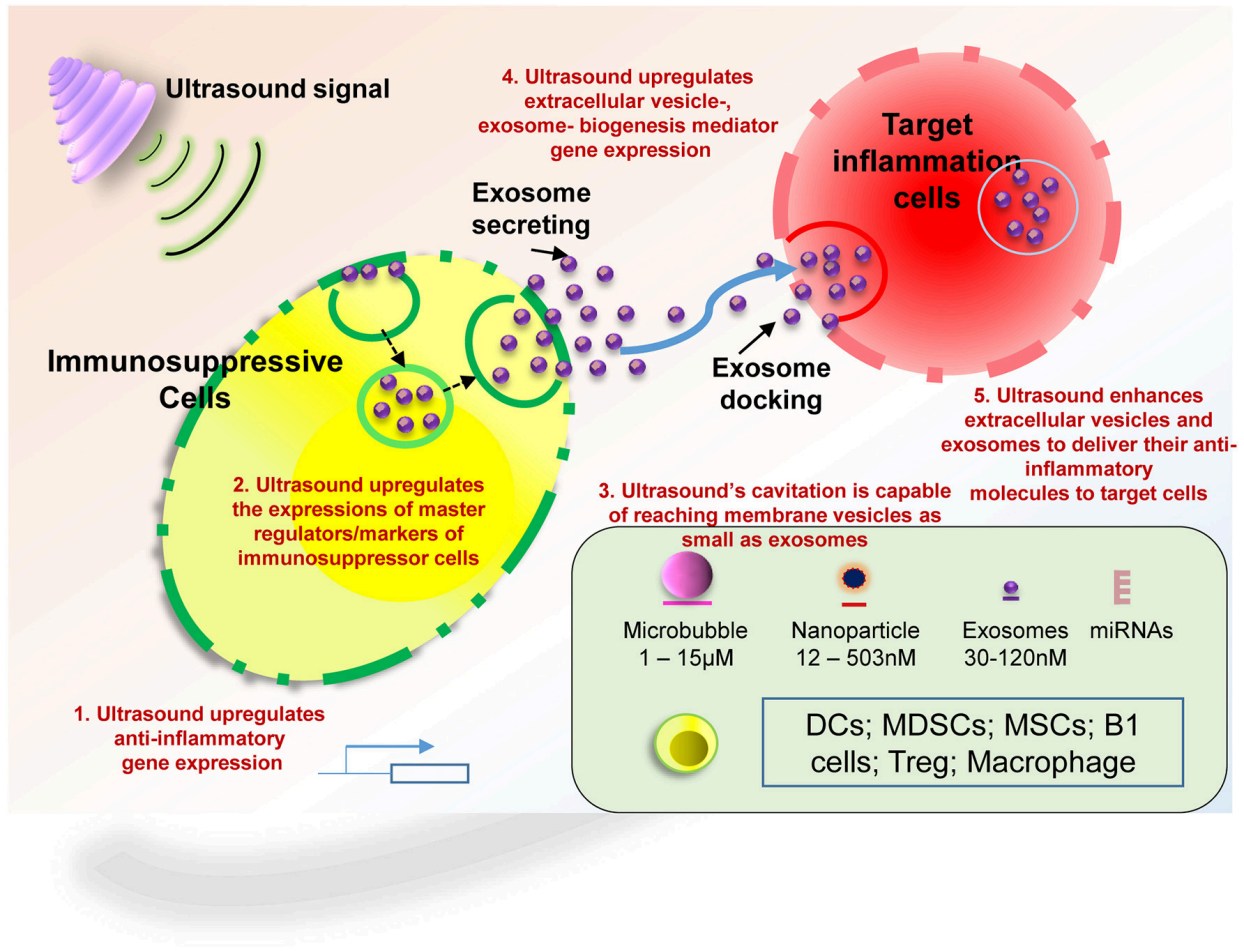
Our novel working model which proposes the potential anti-inflammatory mechanisms utilized by LIUS to exert its beneficial effects. These potential mechanisms are as follows; (1) LIUS upregulates the expression of anti-inflammatory/immunosuppressive genes in the ultrasound-treated cells; (2) LIUS enhances the expression of the master regulators (markers) of immunosuppressor cells; (3) LIUS mediated cavitation is capable of reaching the membrane vesicles as small the expression of extracellular vesicles; and (4) LIUS increases exosome biogenesis and docking mediators, and (5) Ultrasound enhances extracellular vesicles and exosomes to deliver their anti-inflammatory molecules to target cells. Our new findings suggest that LIUS inhibits inflammation via above discussed novel mechanisms to make the target inflammatory cells become less inflammatory. DCs, dendritic cells; MDSCs, myeloid-derived suppressor cells; MSCs, mesenchymal stem cells; B1 cells, CD5+ B1 B cells; Treg, CD4+Foxp3+ regulatory T cells.
